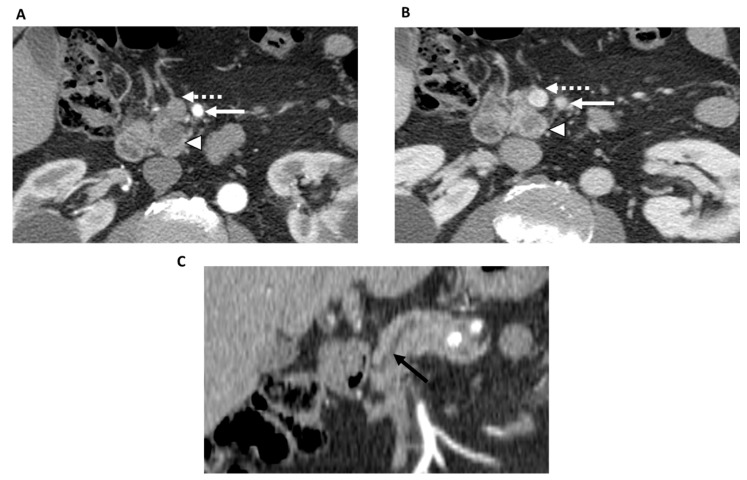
78-year-old man with resectable pancreatic adenocarcinoma within the uncinate process. Contrast-enhanced CT in the axial plane shows a tumor within the uncinate process (arrowhead) in both the arterial (A) and portal venous phases (B). The superior mesenteric artery (white solid arrow) and superior mesenteric vein (dashed arrow) are not involved by the tumor. Contrast-enhanced CT in the coronal plane (C) shows upstream main pancreatic duct dilatation (black arrow). There was no evidence of metastatic disease. Based on the NCCN criteria, the patient was classified as resectable and underwent upfront surgery with R0 resection.
